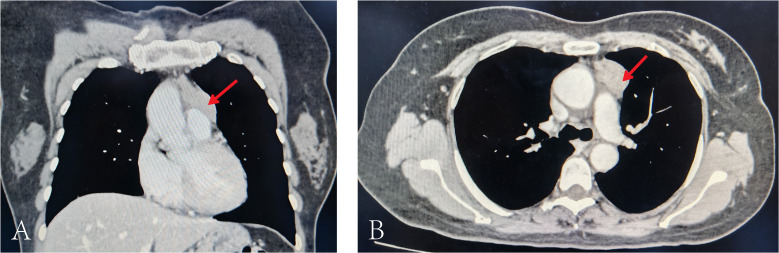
Contrast-enhanced chest CT demonstrating thymoma. (A) Coronal reconstruction. (B) Sagittal reconstruction. Red arrows indicate the anterior mediastinal mass consistent with thymoma.
